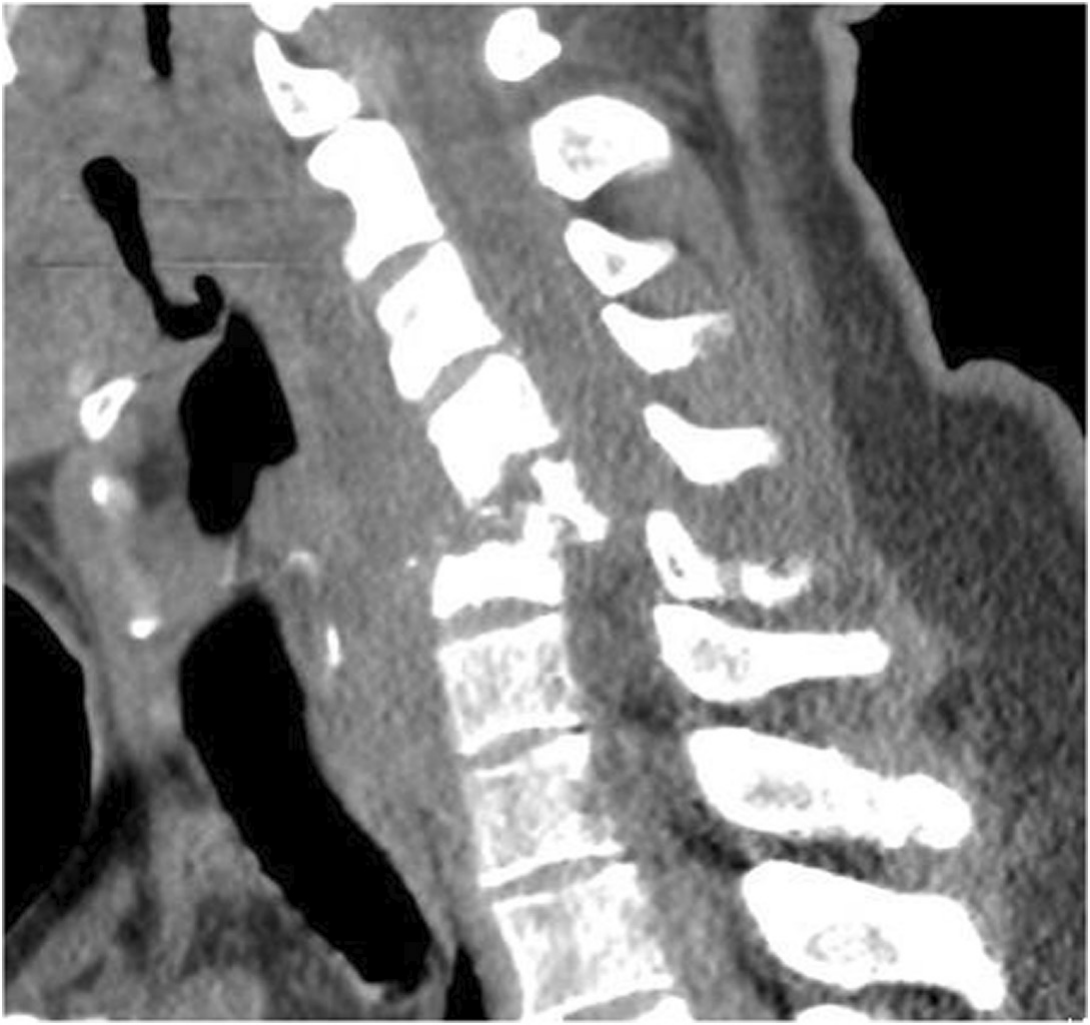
Sagittal CT view showing severe destructive changes at C4‐C5 resulting in severe canal stenosis due to osteomyelitis/diskitis.
